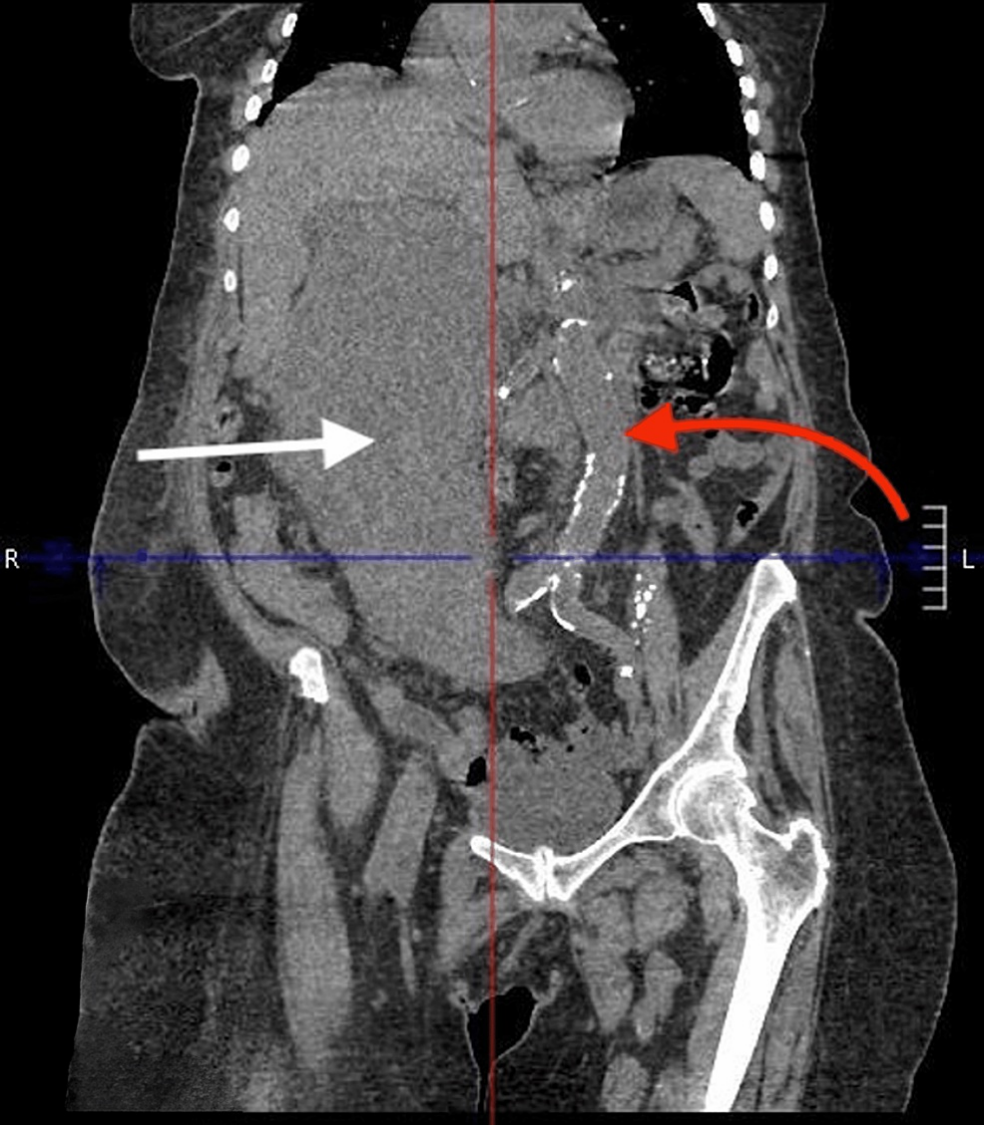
CT abdomen and pelvis CT Abdomen/pelvis coronal plane with the white arrow pointing to the large intraperitoneal mass and the red arrow pointing toward the descending/abdominal aorta being shifted laterally off its medial baseline
